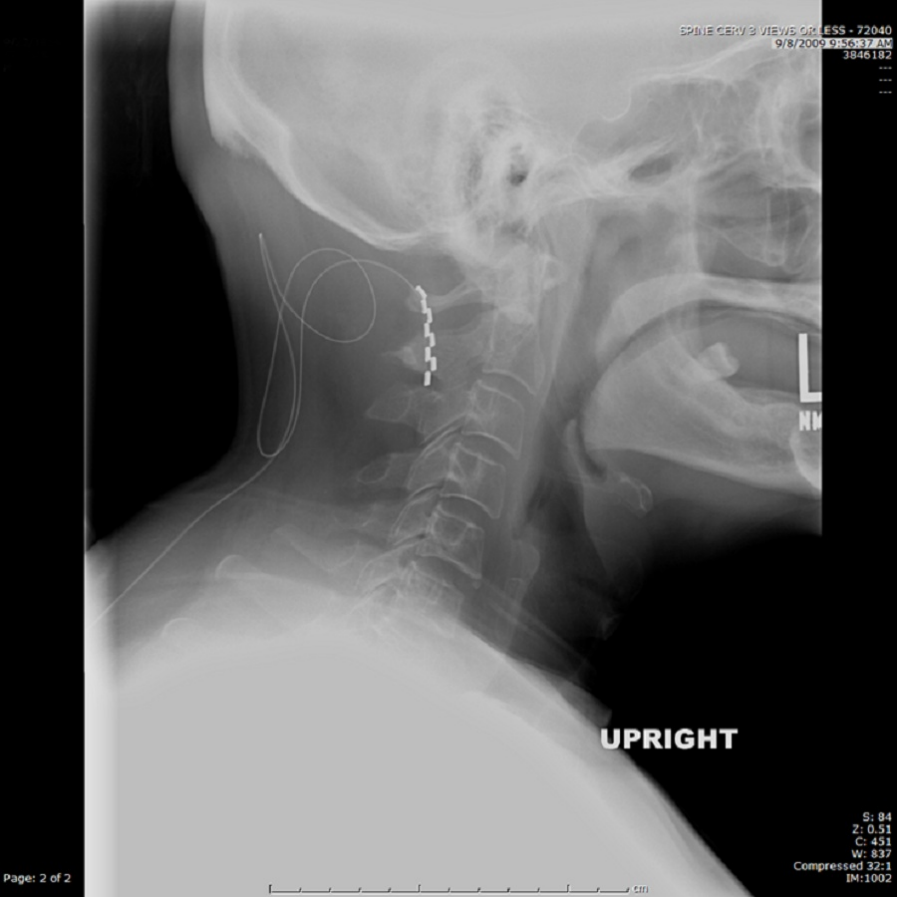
Lateral Cervical Spine X-Ray One-year follow-up image revealing dorsal column stimulator paddle lead at C1-C2
